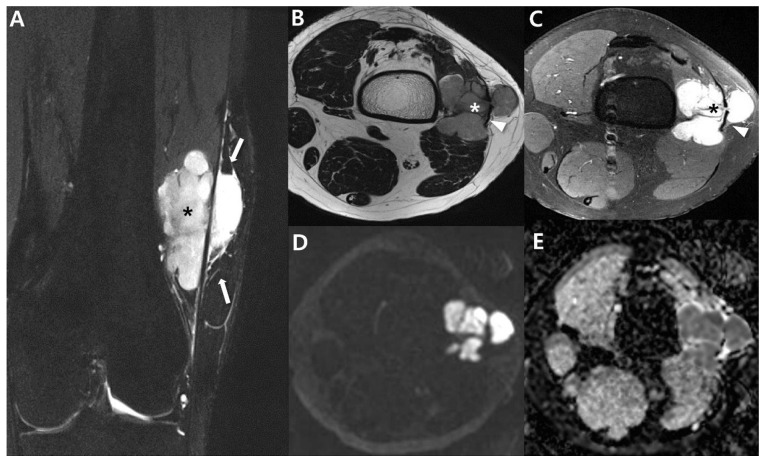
Aggressive features on MRI for large mass of synovial sarcoma of 22-year-old-man (biphasic type): (A) Coronal T2-weighted, fat-suppressed image shows about 3.5 × 3.9 × 5.2 cm sized multilobulated mass with heterogenous signal intensity of Lt vastus lateralis, focally involving biceps femoris muscle. The mass shows presence of T2 hyperintense areas (*) with interfascial (or intercompartmental) extensions (arrows). (B) Axial T2-weighted images show multilobulated, high-signal-intensity mass (*) with extension to subcutaneous fat layer through iliotibial band (arrowhead). (C) Axial T1-weighted, post-contrast image shows well-enhancing mass (*) with intercompartmental extensions (arrowhead). (D,E) Axial DWl (*b* = 1400) and ADC map show high signal intensity and low ADC value, suggesting diffusion restriction.
